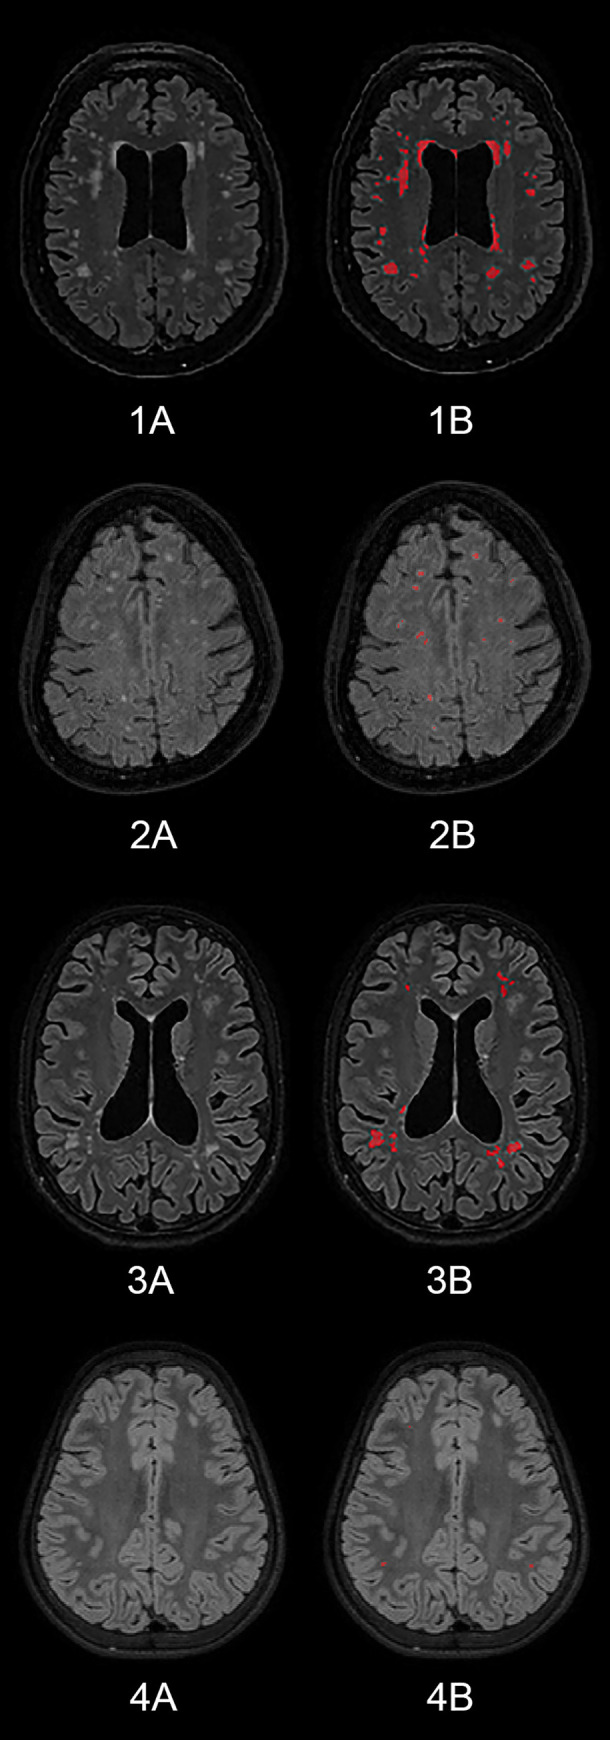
Image segmentations. The images show axial MRI planes of two adults (1 and 2) and two children
